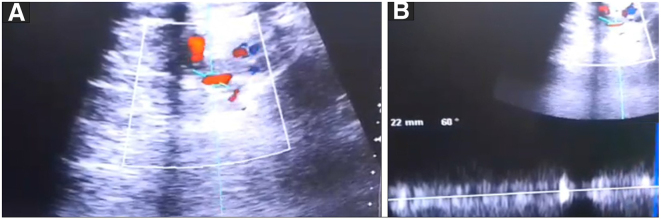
Doppler ultrasound which shows the deep venous insufficiency wave at the level of the left femoral vein.
